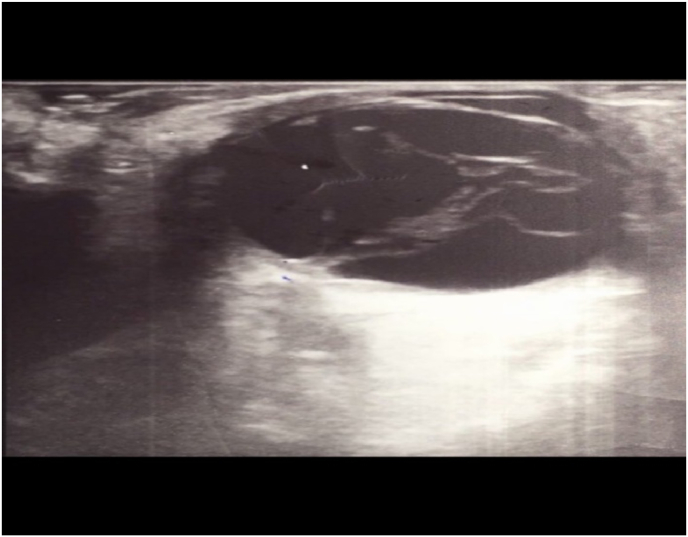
Ocular ultrasound showing a vitreous with an echogenic organization, attached to the detached temporal retinal layer.
